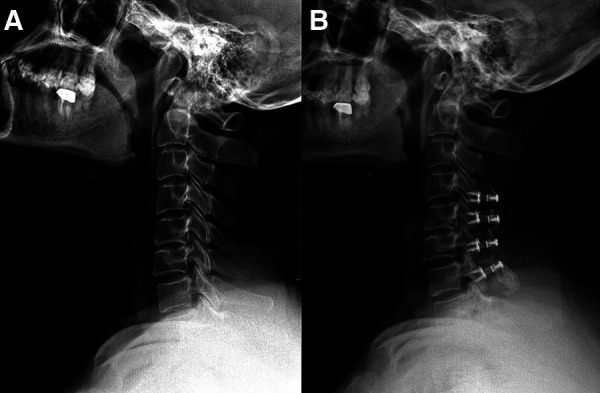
A 60-year-old male patient treated with C4–C7 laminoplasty. (A) Preoperative lateral cervical radiograph (CSVA = 9.7 mm, T1S = 20.7°). (B) Lateral cervical radiograph at final follow-up (19 months after surgery, CSVA = 11 mm).
